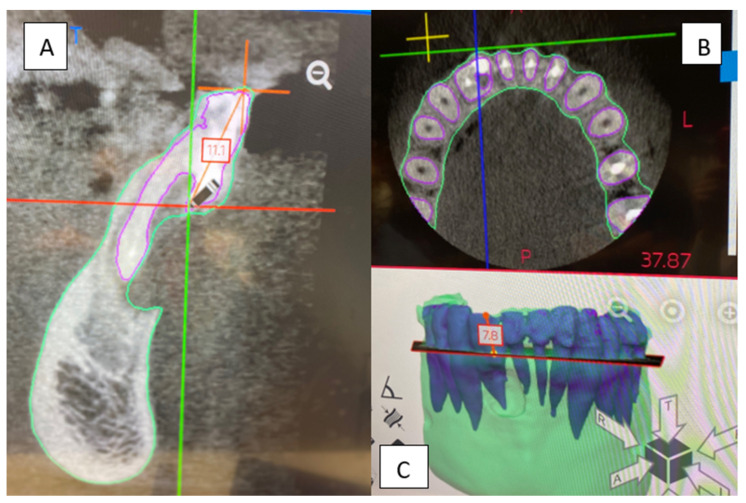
CBCT sagittal (A) and axial (B) sections of tooth #26. (C) Measurements taken from the CBCT sections and 3D software aided in accurate non-surgical and surgical planning. Axial section apically showing dilated space of DI.
